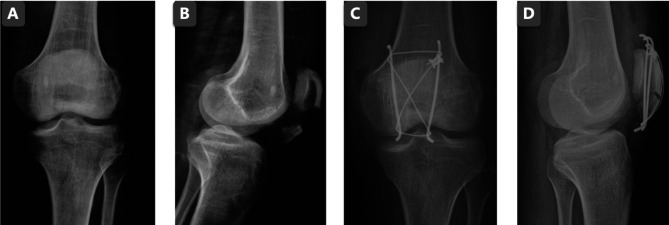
The AP knee joint X-ray before the surgery (A); the lateral knee joint X-ray before the surgery (B); the AP knee joint X-ray post the traditional method surgery (C); the lateral knee joint X-ray post the traditional method surgery (D).
